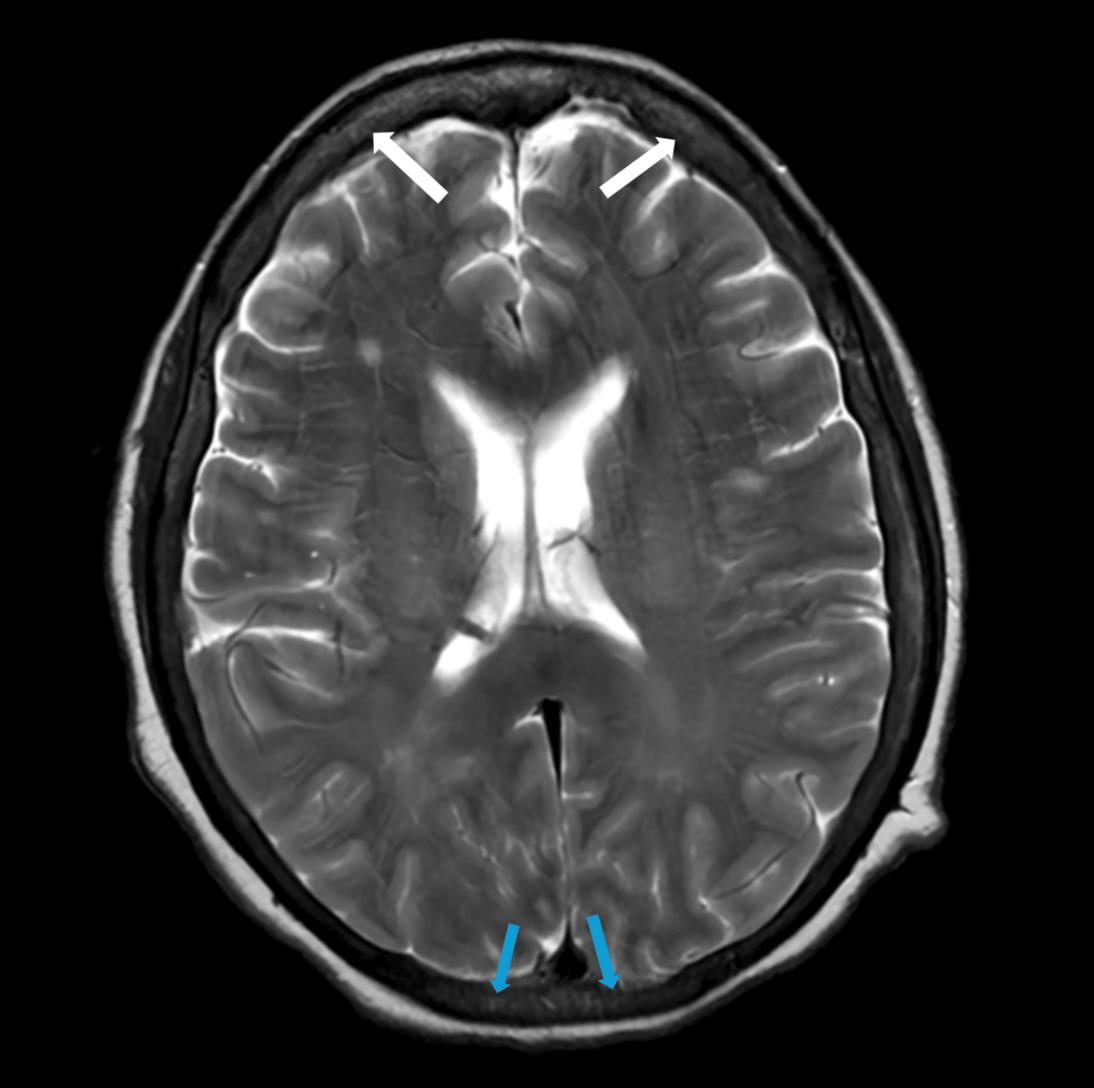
MRI brain imaging showing bilateral frontal and posterior fossa subdural hematomas White arrows point to the bilateral frontal subdural hematomas. Blue arrows point to the bilateral posterior subdural hematomas.
